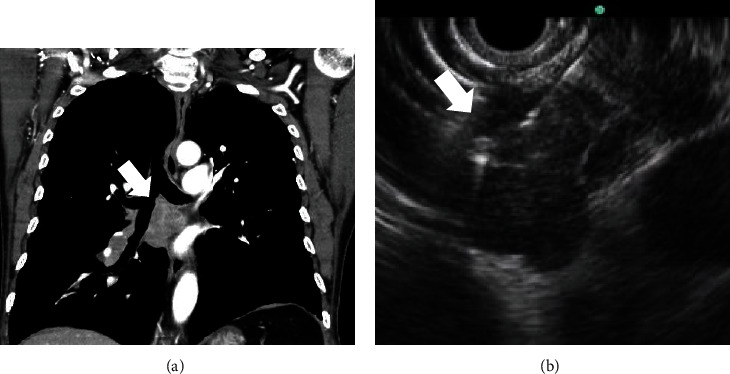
A 78-year-old male patient. (a) Subcarinal lymphadenopathy (arrow) is shown on CT scan. (b) On EUS view, multiple round shaped and sharp-demarcated lymph nodes (arrow) were conglomerated in station 7. The EUS-guided biopsy was performed. CT: computed tomography; EUS: endoscopic ultrasound.
